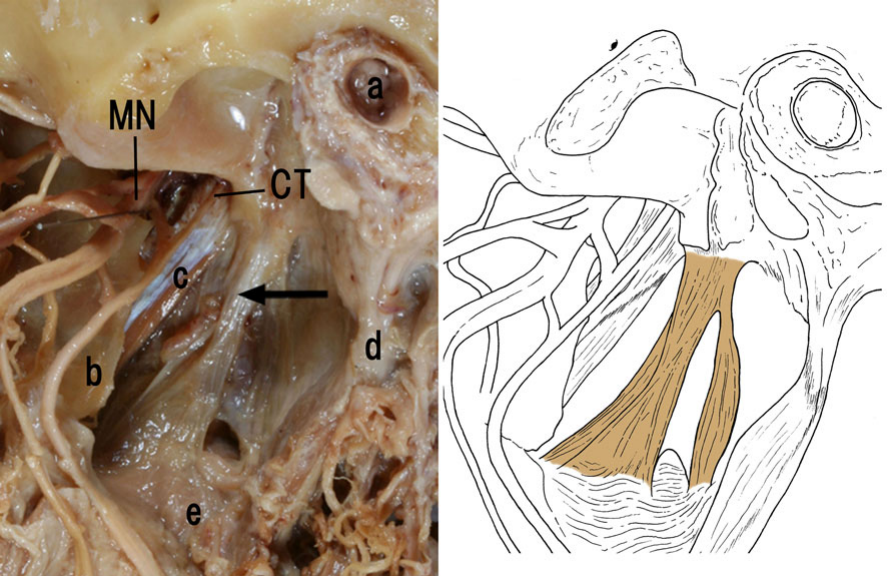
Type A. Left side appearance of the infratemporal fossa of a 75-year-old Japanese woman. *Arrow* Previously unidentified tissue. *CT* Chorda tympani, *MN* mandibular nerve. *a* External acoustic opening, *b* lateral plate of pterygoid process, *c* tensor veli palatini, *d* styloid process, *e* superior constrictor
